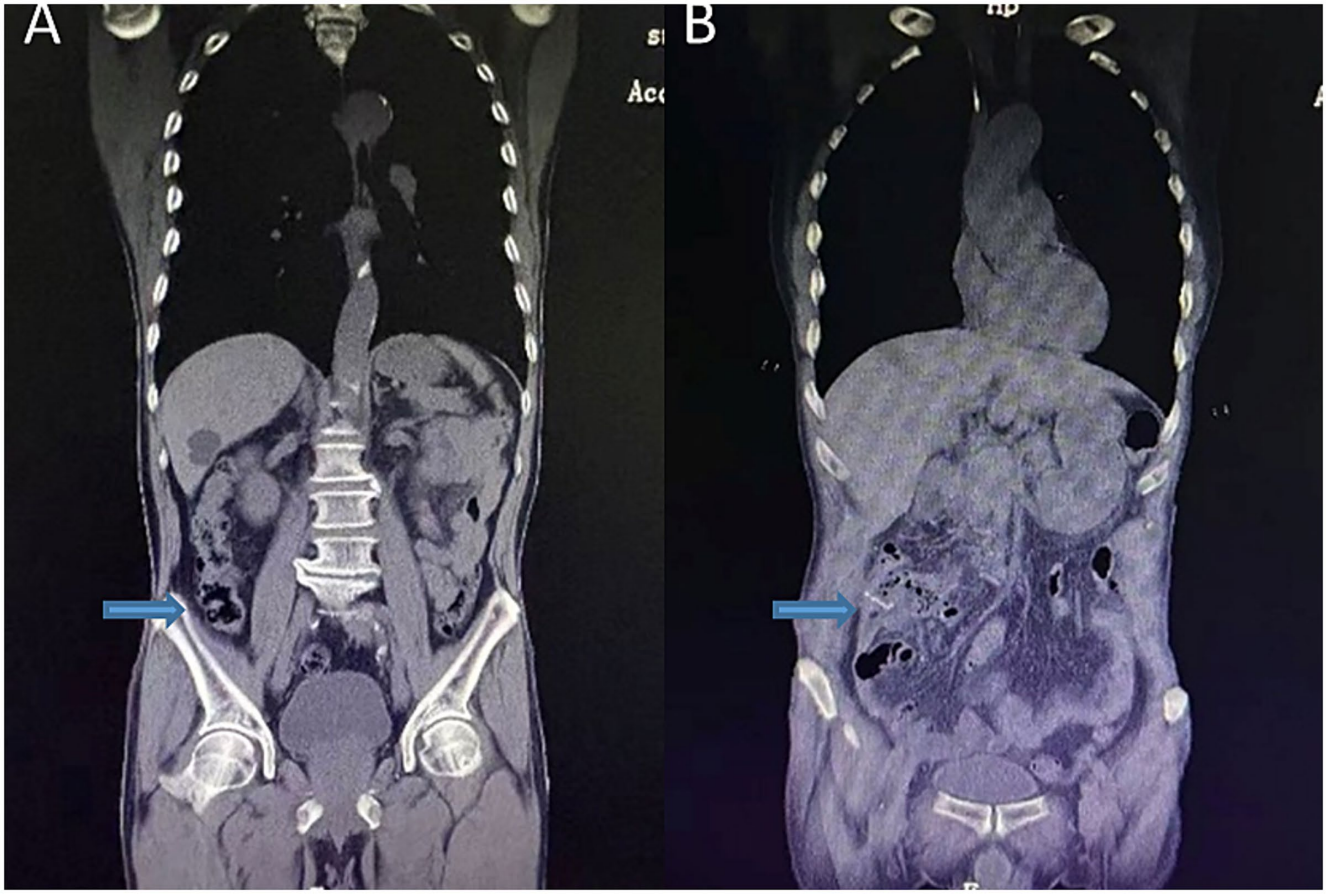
Preoperative abdominal coronal view (A); postoperative abdominal CT coronal view (B). The arrows indicate preoperative localized bowel wall thickening and the postoperative anastomosis.
